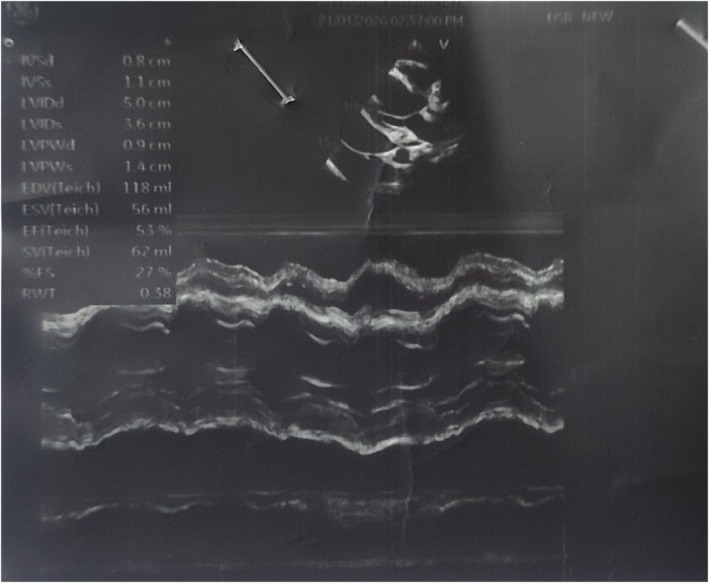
Echocardiography showing Cardiac Tamponade.
